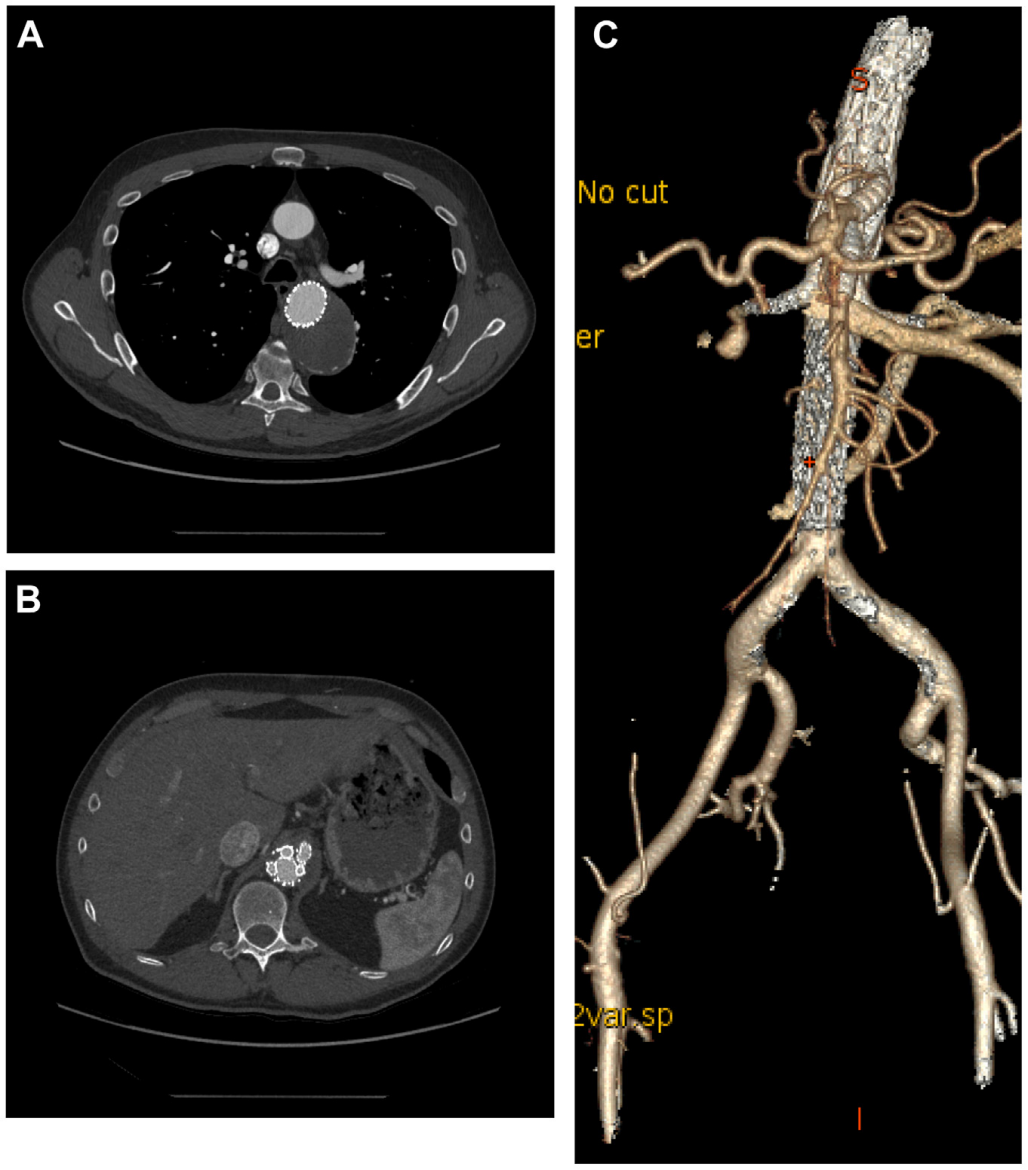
Postoperative images. (A) Complete thrombosis of the aneurysm at the proximal descending aorta. (B) Multi-branch stent graft at the level of the celiac artery. (C) Three-dimensional (3D) reconstructed image of the computed tomography (CT) angiogram.
